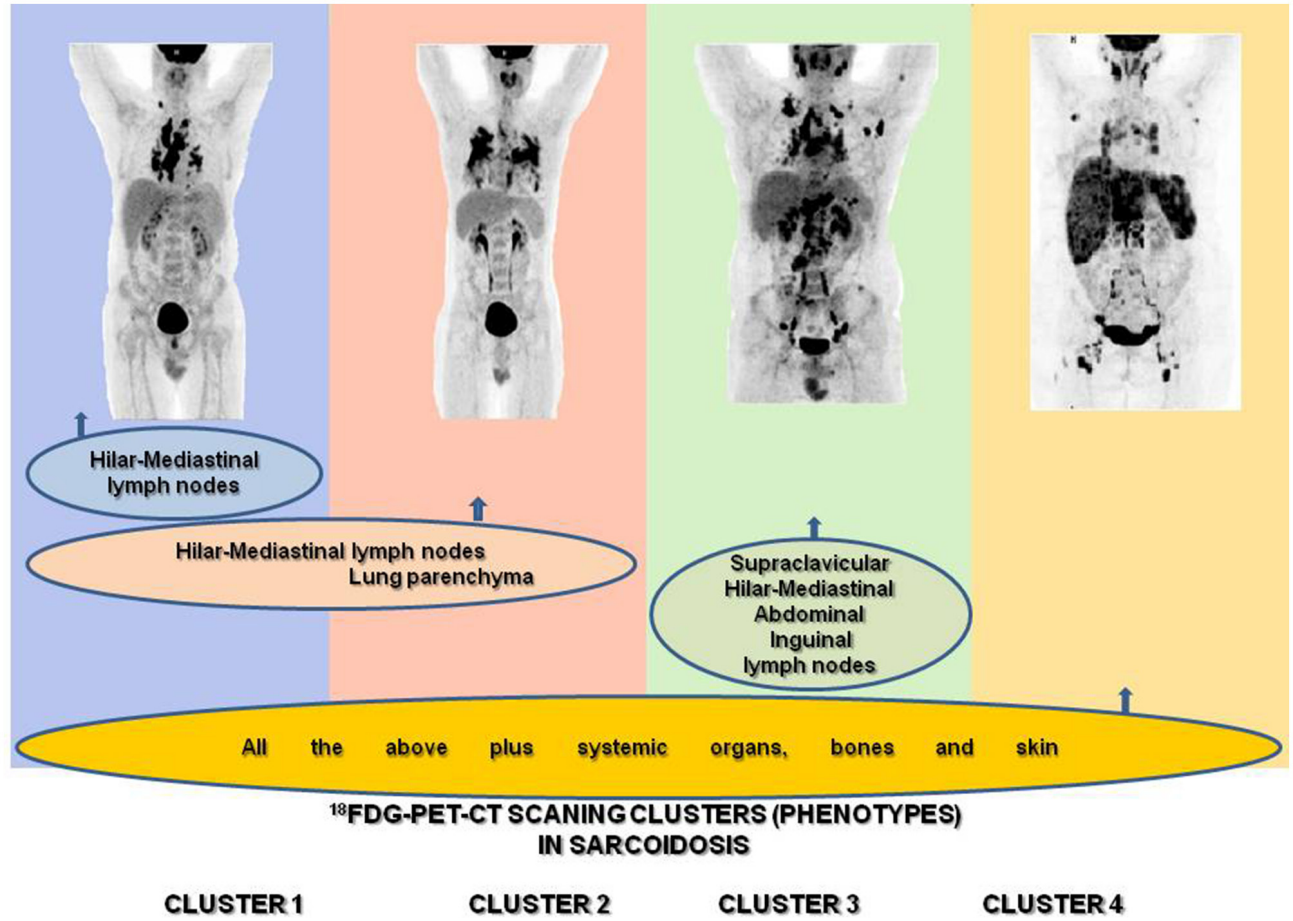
Identification of sarcoidosis ^18^F-FDG PET/CT scan phenotypes according to organ involvement using two-step cluster analysis: (1) hilar–mediastinal nodal, (2) lungs and hilar–mediastinal nodal, (3) an extended nodal supraclavicular, thoracic, abdominal, inguinal, and (4) all the above in addition to systemic organs and tissues such as muscles–bones–spleen and skin.
